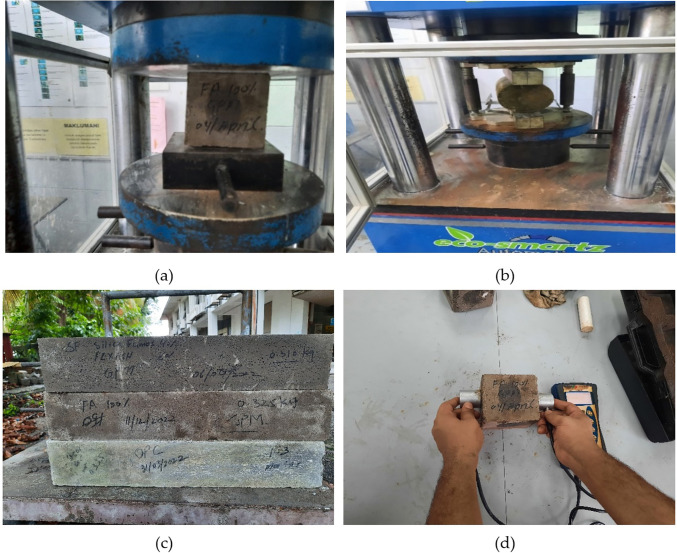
Experimental testing setup for FA and SF-based GP-Mortar: (a) Compressive strength; (b) Splitting Tensile Strength; (c) Residual Flexural; (d) Ultrasonic pulse velocity (UPV).
